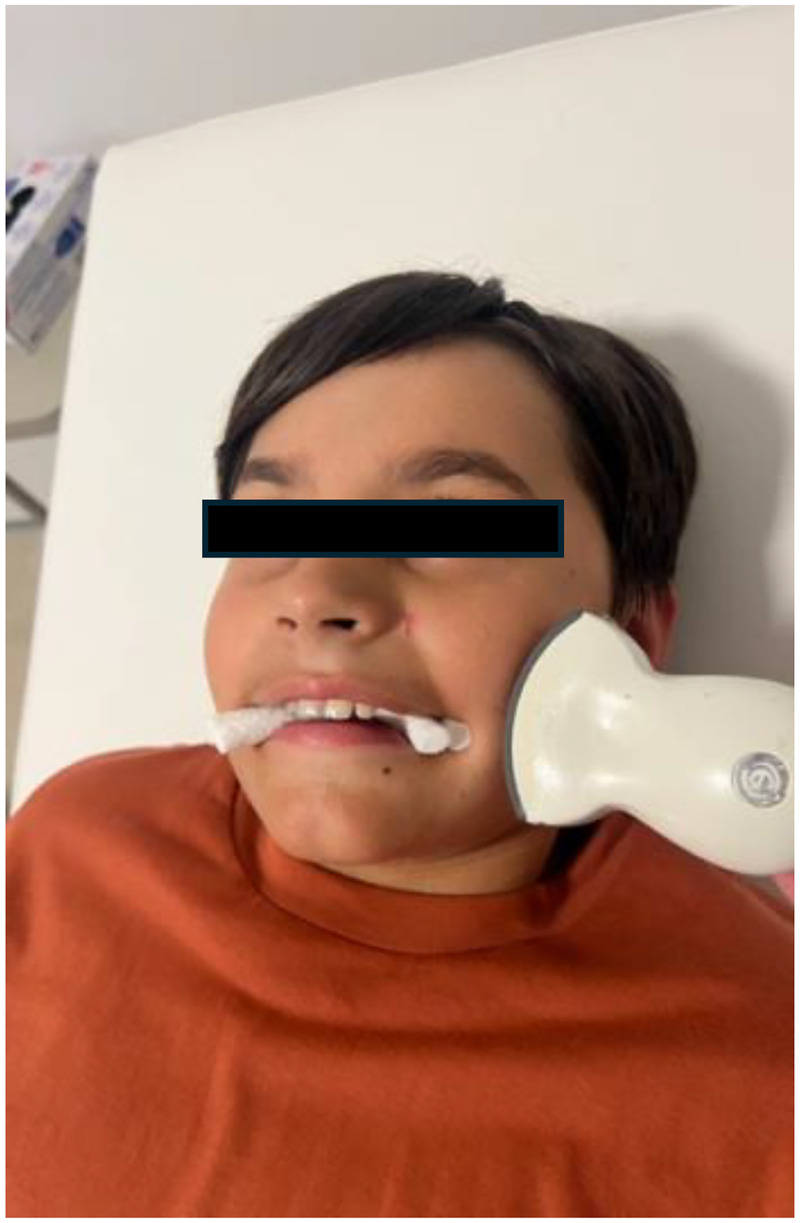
Ultrasound examination of the muscle (N.M., 9 y.o., male).
